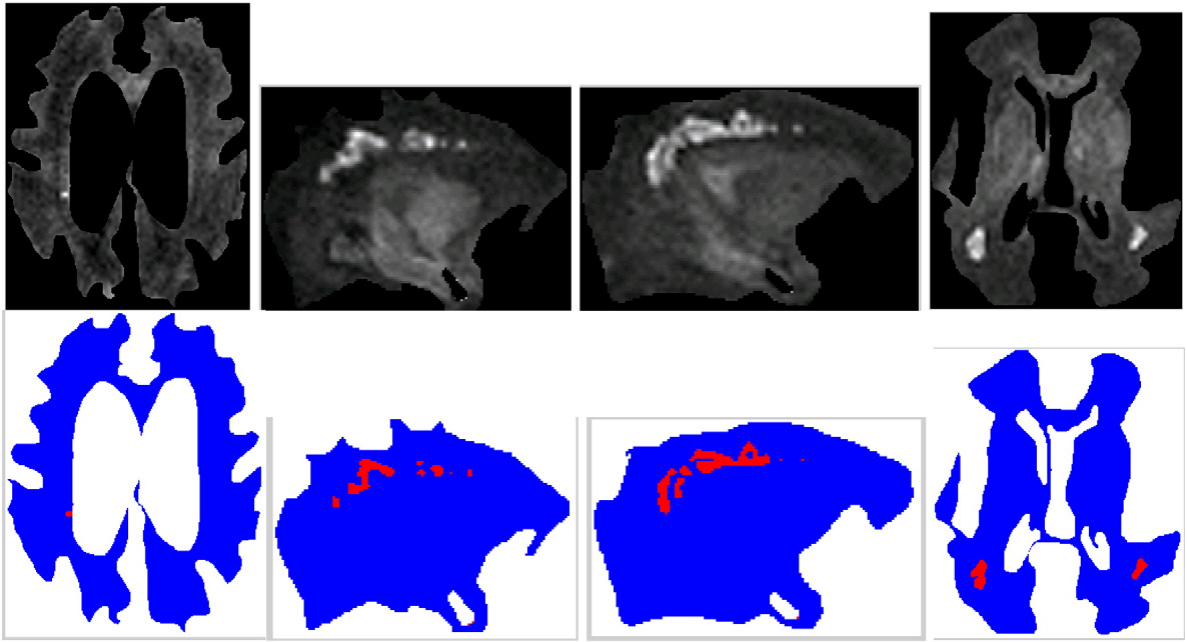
(Top row) white matter regions in MRI slices after pre-processing; and (bottom row) injuries detected automatically (marked in red) by our stochastic algorithm.
